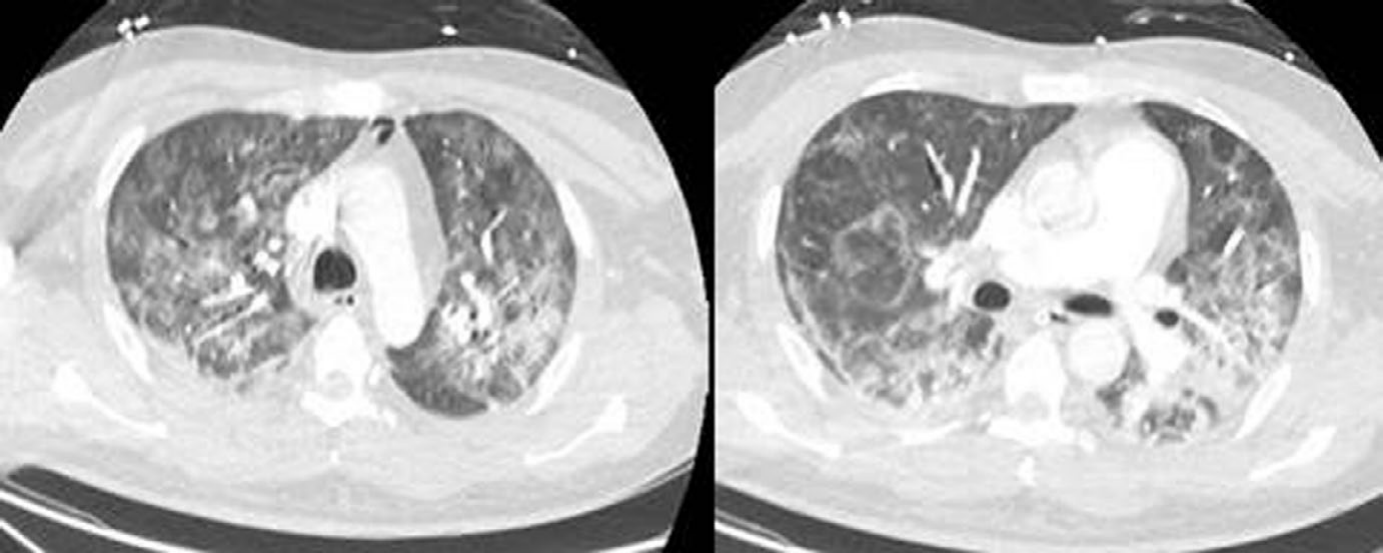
Contrast chest computed tomography scans depicting peripheral bilateral ground‐glass opacities, especially on the right lung, in our patient with severe COVID‐19
